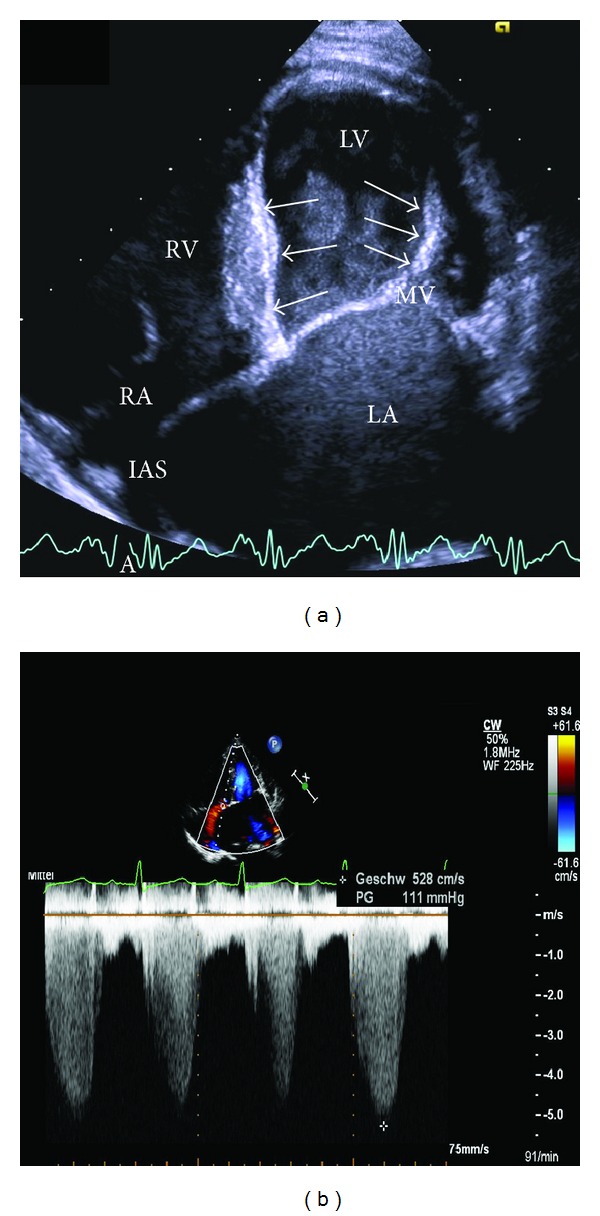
Echocardiography: 4-chamber view showing extremely enlarged left atrium (LA) and endocardial fibroelastosis (arrows) of left ventricle (LV) and mitral valve apparatus (a). Doppler maximum velocity of tricuspid insufficiency before initiation of medical therapy, indicating high systolic RV pressure of 111 mmHg (b). RV = right ventricle; RA = right atrium; IAS = interatrial septum.
